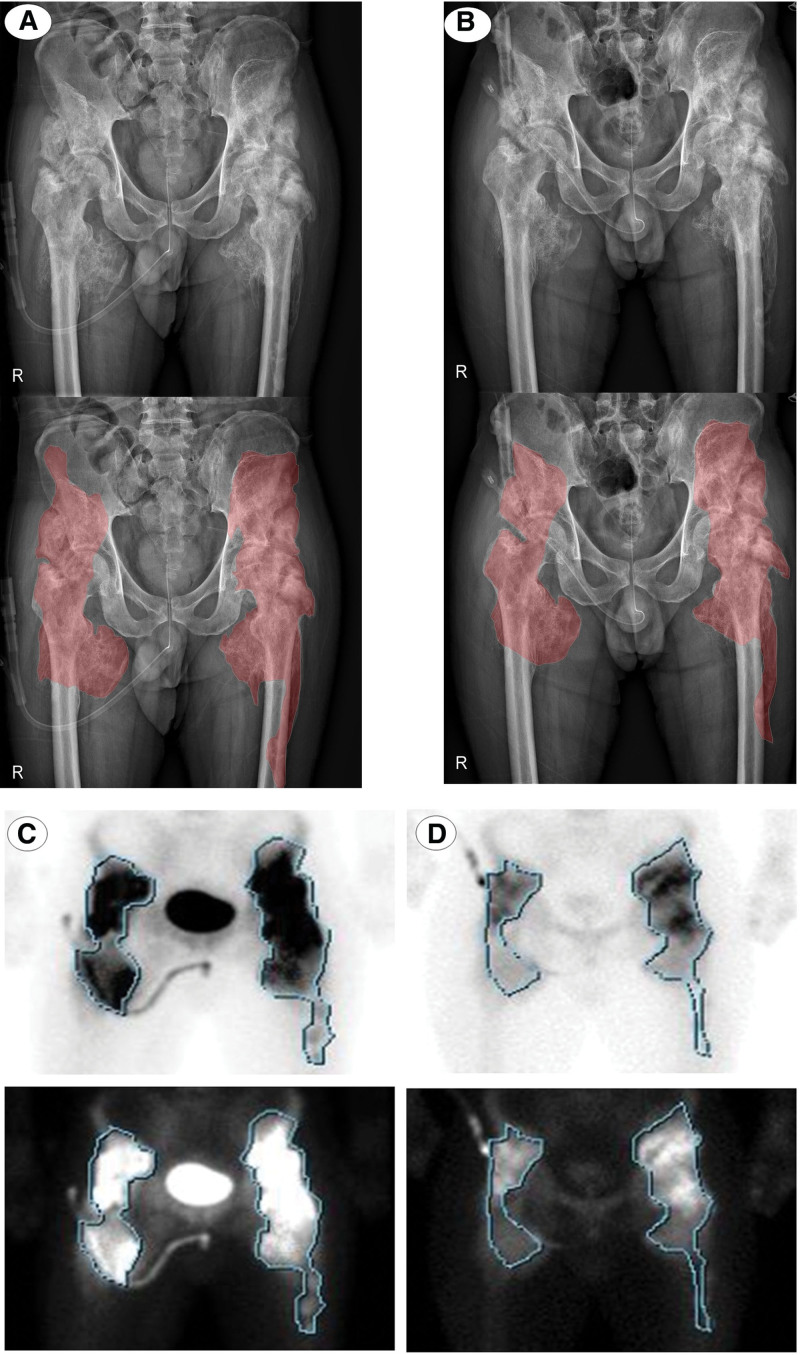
Changes in radiologic findings before and after ESWT treatment: (1-A) Plain X-rays of both hips before (A) and after (B) ESWT (above: raw image; below: with contour of HO); (1-B) Three-phase bone scan before (C) and after (D) ESWT (above: raw image with contour of HO; below: color inverted to show clear boundary of HO). ESWT = extracorporeal shock wave therapy, HO = heterotopic ossification.
